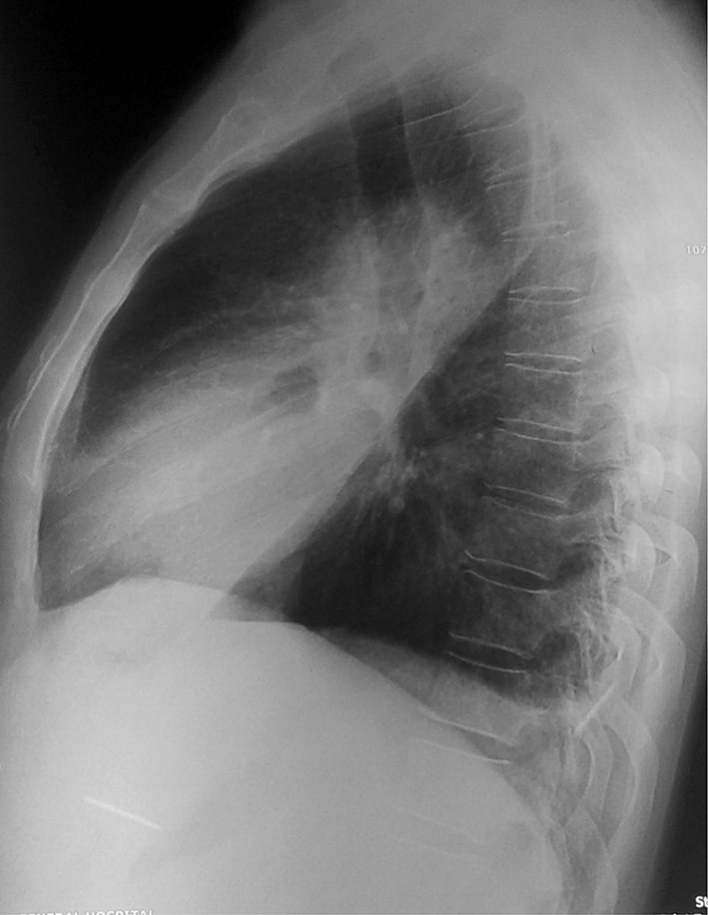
Plain x-ray of chest, left lateral view (on admission)
